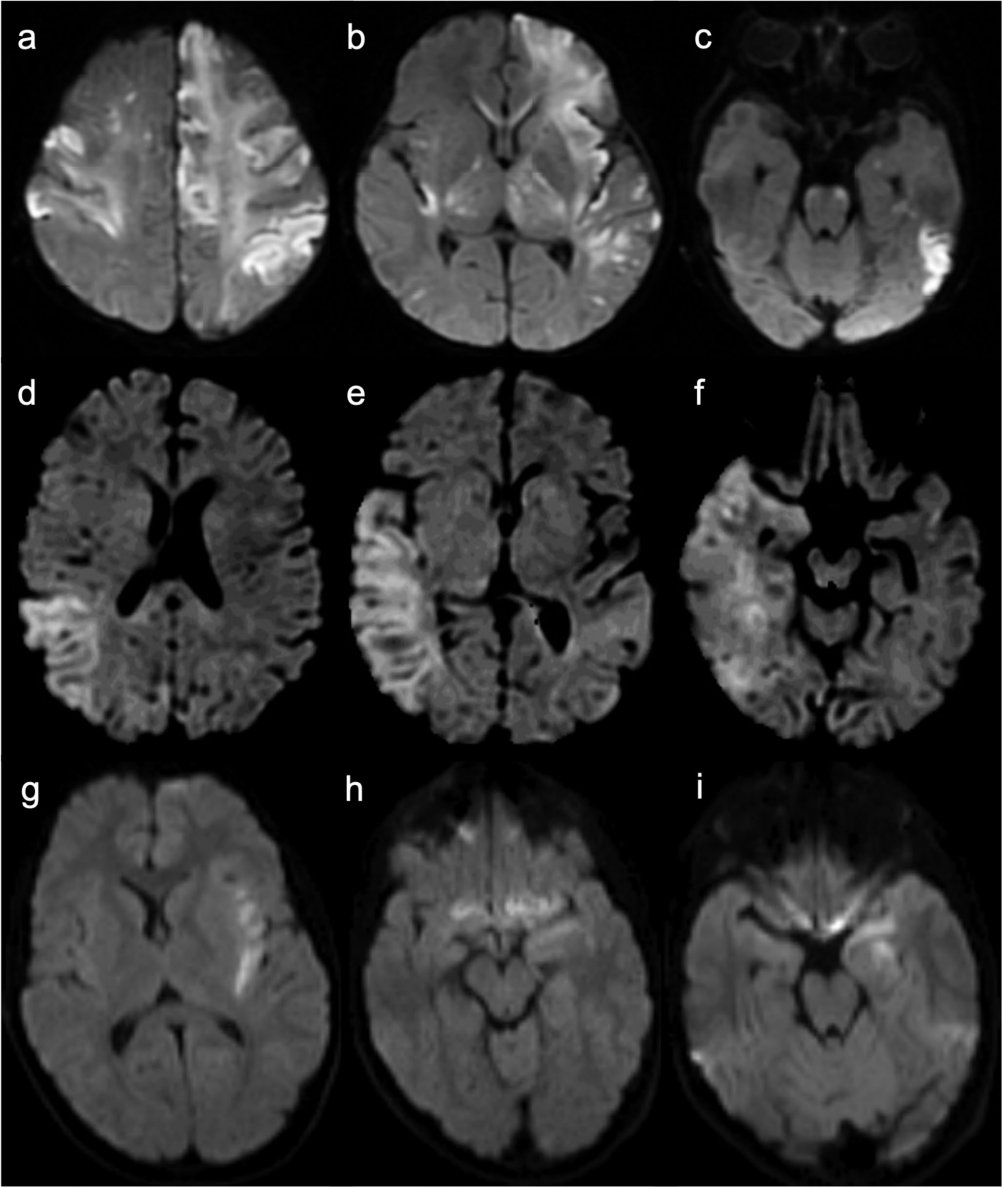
Diffusion MR imaging patterns in Herpes simplex encephalitis. (a to c) Neonatal HSV type 2 encephalitis in a 20-day-old male patient. Multifocal, asymmetric areas of DWI hyperintensity (corresponding low signal on ADC maps is not shown) involving cortical and subcortical regions, including the corpus callosum, thalami, posterior limbs of the internal capsules, and anterior pons. (d to f) HSV type 1 encephalitis in a 12-month-old female patient. Bilateral asymmetric cortico-subcortical lesions with DWI hyperintensity, predominantly in the right temporoparietal region, mimicking a right middle cerebral artery infarction. (g to i) HSV type 1 encephalitis in an 11-year-old male patient. Predominantly left-sided cortical lesions with DWI hyperintensity involving the medial temporal lobe, insula, and frontal cortex; subtle frontobasal involvement on the right. Cortical hemorrhagic foci also present (not shown)
